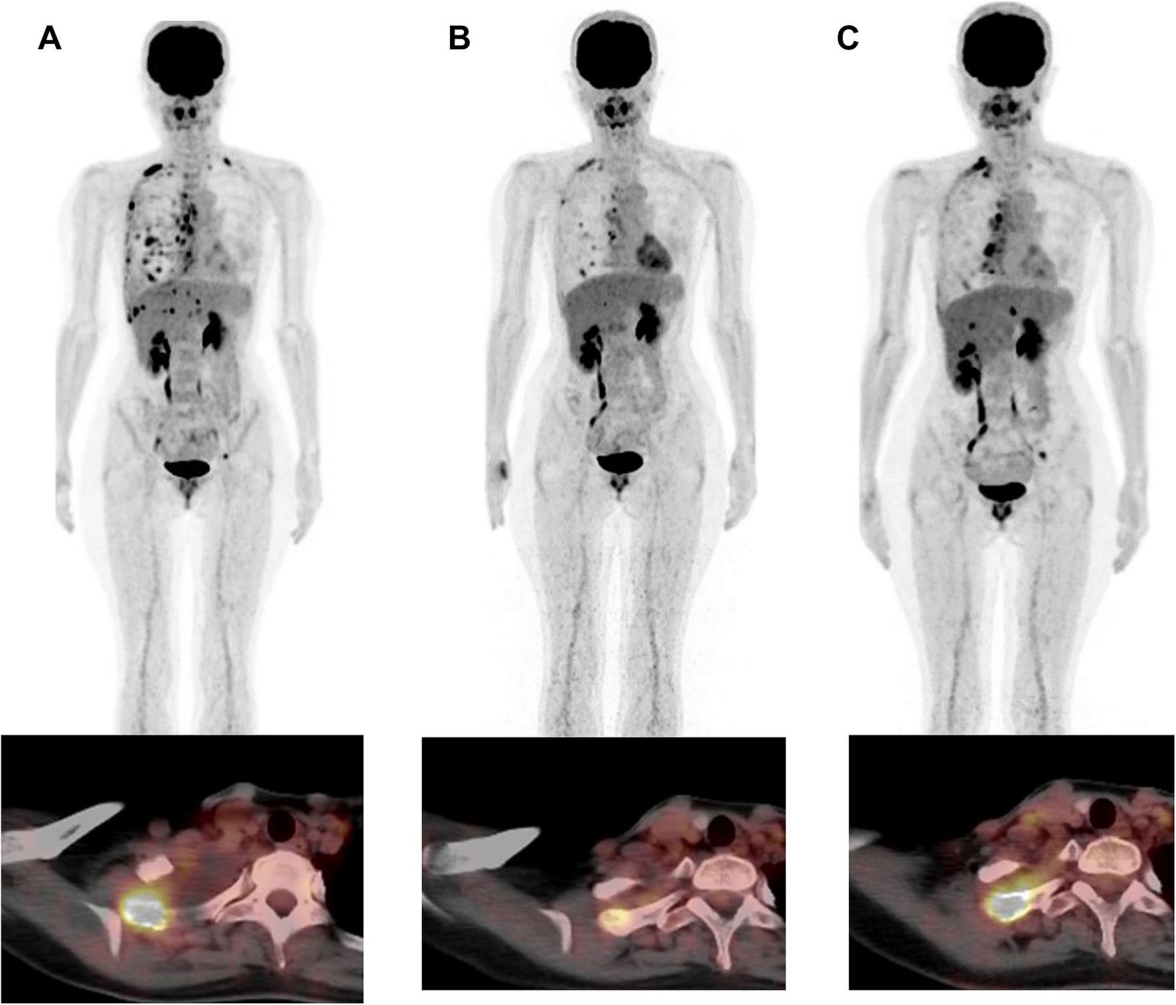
This is Case No. 11 in Table 6. Anterior views of FDG–PET MIP images, and FDG–PET and CT fusion axial images at the right first rib are shown. She noticed shortness of breath 10 years after her right breast surgery. FDG–PET/CT was performed, and pleural dissemination and multiple osteolytic skeletal metastases were diagnosed (a). She was prescribed hormone therapy after the FDG–PET/CT study (a). First follow-up FDG–PET/CT was performed after 6 months of hormone therapy and showed improvement in pleural dissemination and skeletal metastases (b). Because her tumor marker (CA15-3) was elevated (42.3 U/ml), second follow-up FDG–PET/CT was performed. FDG uptake in skeletal metastasis has increased and was judged to be progressive disease (c). Her initial WS-TLG value was 15.5, and the value was 0 at first follow-up and 4.6 at second follow-up FDG–PET/CT study
